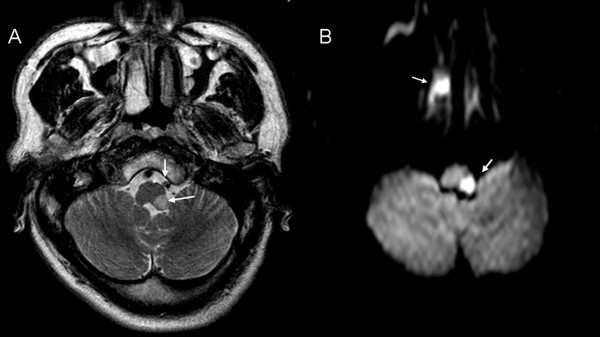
MRI of the patient: (A) Axial T_2_-weighted image showing hyperintense signal in the left lateral medullary and narrow lumen of the left vertebral artery; (B) Diffusion-weighted image showing hyperintense signal in the left lateral medullary and hyperintense signal of left inferior turbinate.
